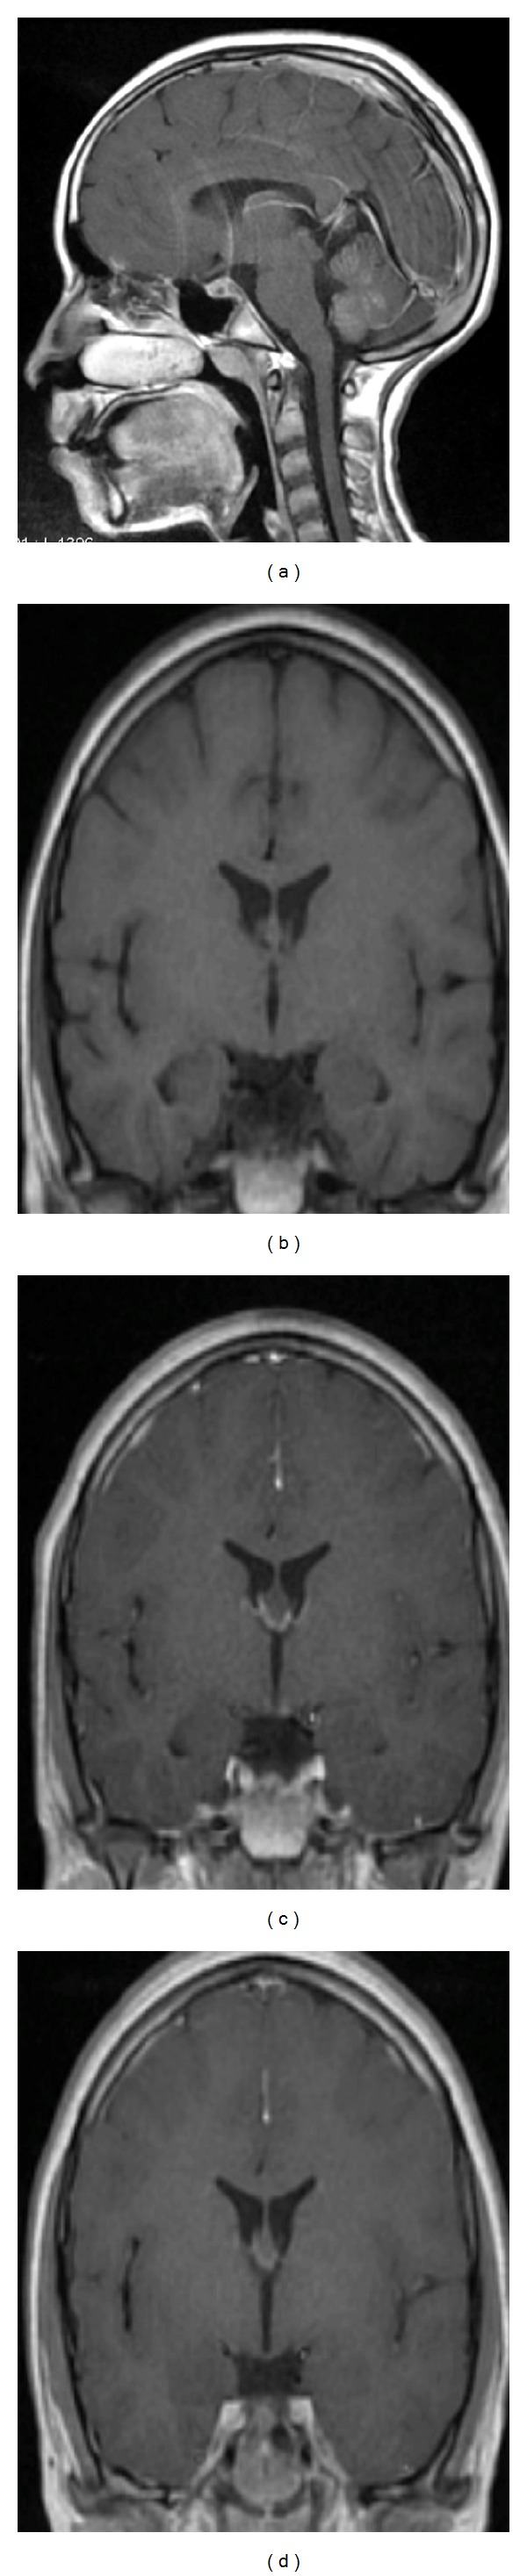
(a) Sagittal T1 with contrast image showed persistence of gadolinium in a follow-up study conducted 4 months later. (b)–(d) Sequential coronal T1 images showing the baseline mamillary body when MRI was performed during the first episode (b), enhancement of mamillary body during the second episode (c), and atrophy of mamillary body in a follow-up MRI performed 4 months later (d).
